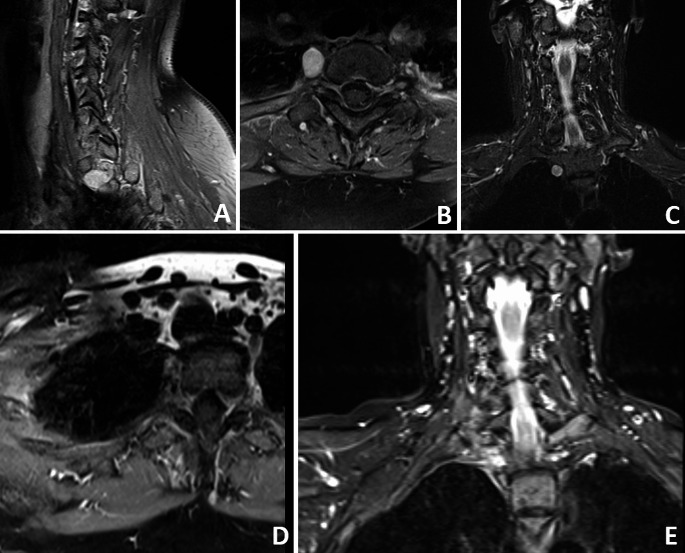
Exemplary case of a completely paraspinal schwannoma (case 29). The tumor mass is in contact with the spine as well as the apical lung as shown in the sagittal (A), axial (B), and coronar (C) MRI scans. GTR was achieved as the follow up MRI shows in axial (D) and coronar (E)
